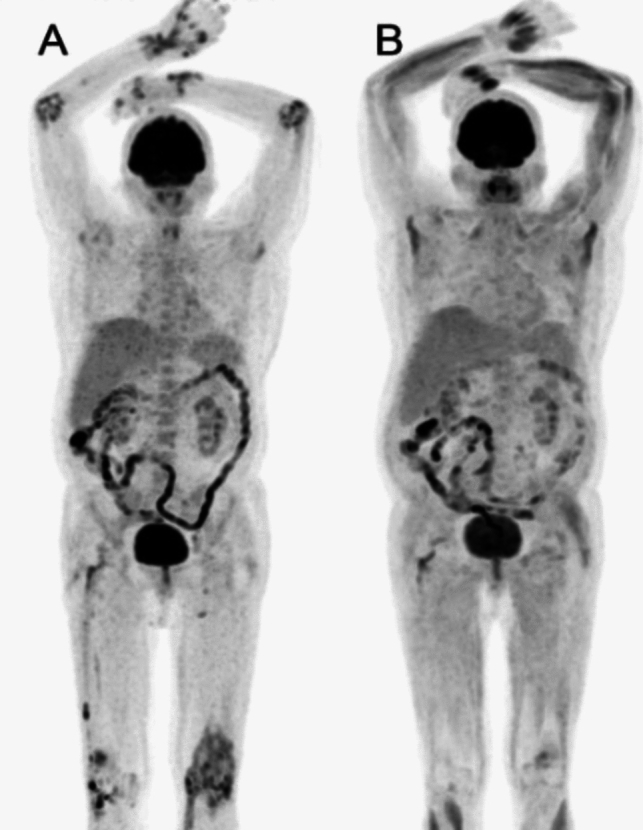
The study examined joint inflammation using [^18^F]-FDG PET/CT in a patient with rheumatoid arthritis at baseline (A) and after 12 months of tofacitinib treatment (B). The maximum intensity projection PET/CT image at baseline (A) displayed increased synovial activity in multiple joints, including the wrists, small hand joints, elbows, and knees bilaterally. However, in the follow-up image (B), after 12 months of tofacitinib treatment, there was a decrease in [^18^F]-FDG uptake, indicating a reduction in joint inflammation. With permission from reference [60]
